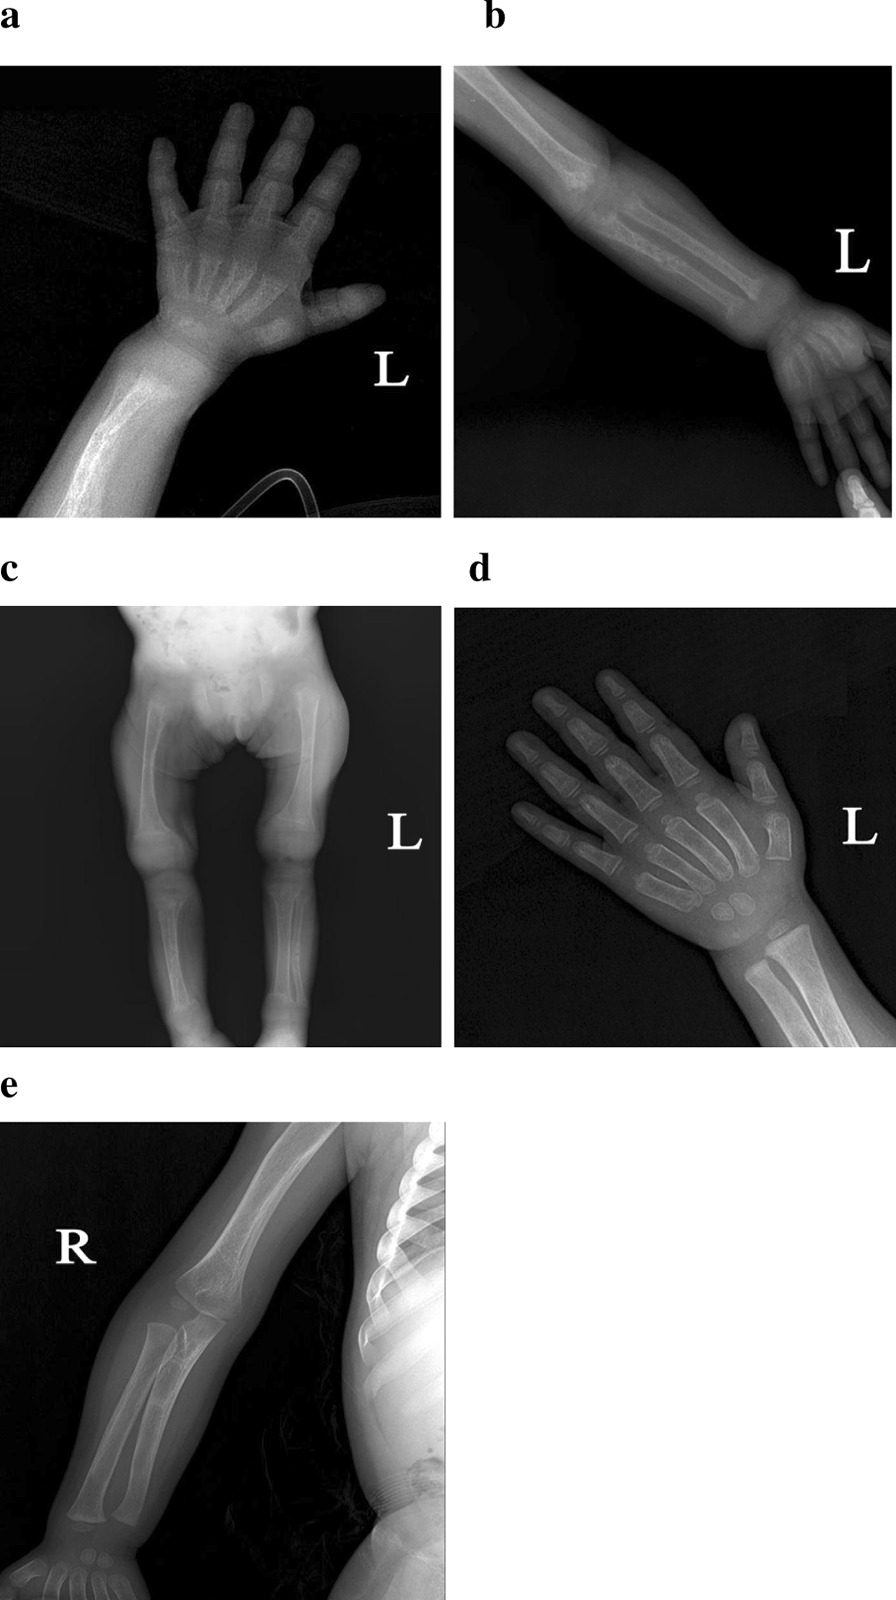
Photographs and radiographs of the patient 2 with VDDR-IA. a Before treatment, wrist radiograph disclosed the vanishing of the zone of provisional calcification in distal metaphysic, widened metaphysic and left radius fracture. b Before treatment, right ulna fracture with callus formation. c Before treatment, lower limbs has thinness of the cortical bone, rarefaction of bone trabecula, widened, frayed, and irregular metaphysis in the long bones, and soft tissue swelling around the wrist joints. d After 1 year of treatment, wrist radiograph revealed fracture healing and reoccurrence of the zone of provisional calcification in distal metaphysis, along with improvement of bone trabecula and thickening of the cortical. e After 1 year of treatment, right ulna fracture showed healing
